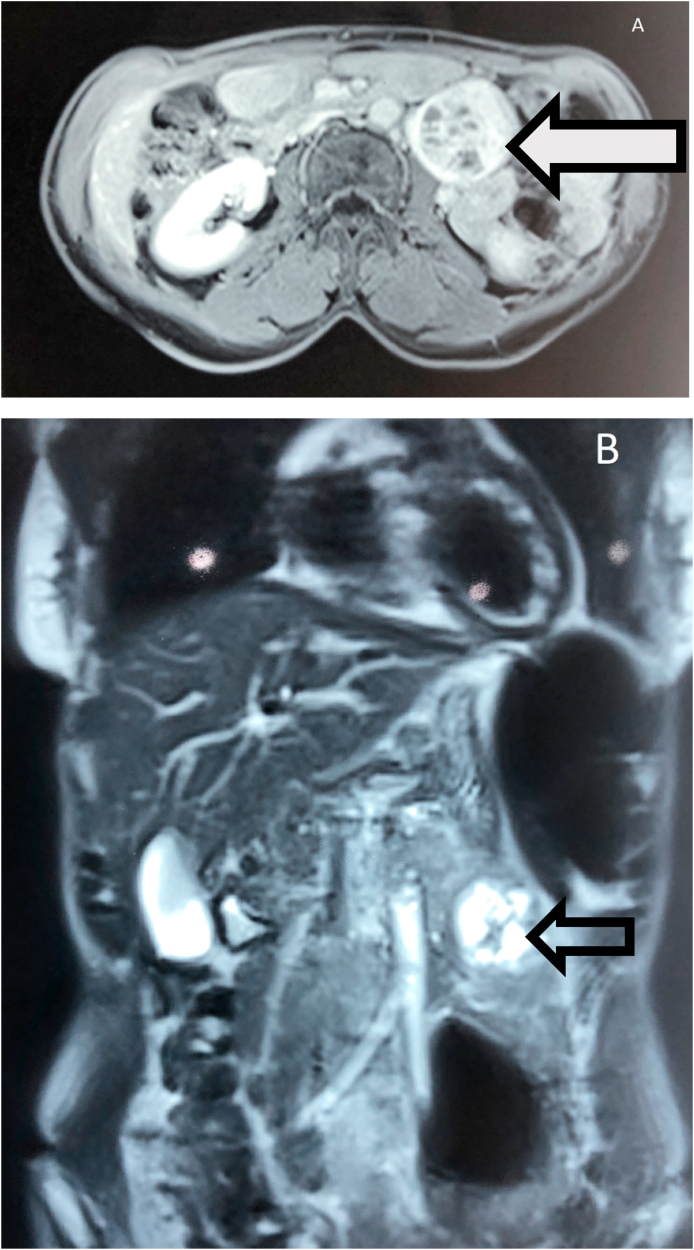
(A, B) Contrast magnetic resonance imaging (MRI) of the abdomen (axial and coronal planes) revealing a well-defined Left latero-aortic cystic retro-peritoneal mass (left arrow).
